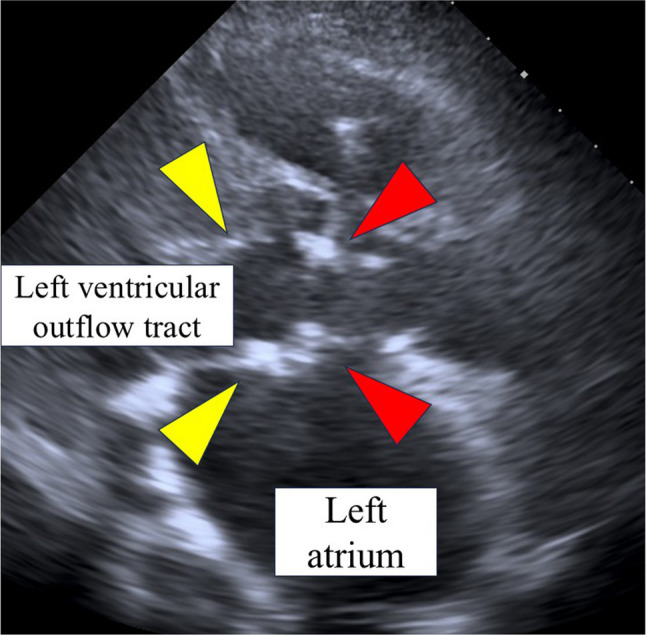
Transthoracic echocardiography before emergent surgery. Transthoracic echocardiography demonstrates the left ventricular outflow tract (yellow arrowheads) and the prosthetic aortic valve (red arrowheads). A clear separation between the prosthetic valve and the surrounding tissue is observed, indicating prosthetic valve dehiscence. These findings are highly suggestive of prosthetic valve endocarditis with annular involvement
